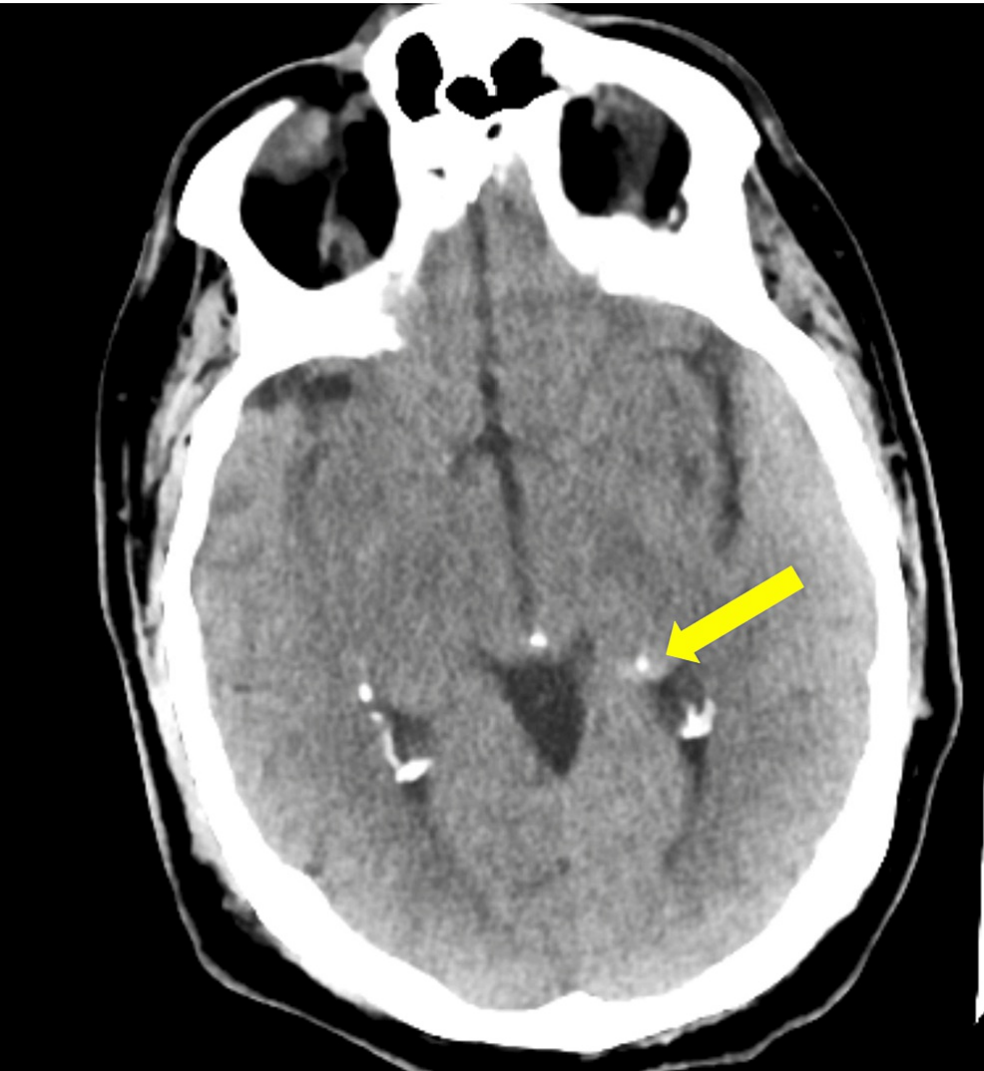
CT head showing hyperattenuation in the left hippocampus suggestive of calcification and/or mineralization. CT, computed tomography
